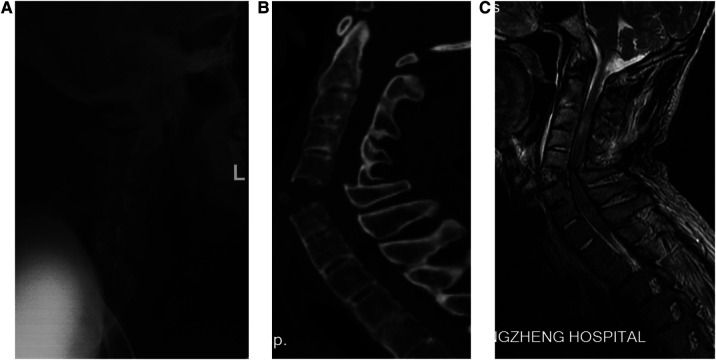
A 57-year-old man with AS and thoracic kyphosis and was diagnosed as fracture dislocation at C5/6. (A) Lateral radiography reveal a severe dislocated fracture at C5–6 levels with significant widen at C5–6 intervertebral space and so-called bamboo spine resulting from ankylosing spondylitis. (B) Sagittal CT showing fracture dislocation at C5/6. (C) MRI demonstrating increased signal segmentally at the level of the fracture indicate total segmental disruption. AS, ankylosing spondylitis; MRI, magnetic resonance imaging.
